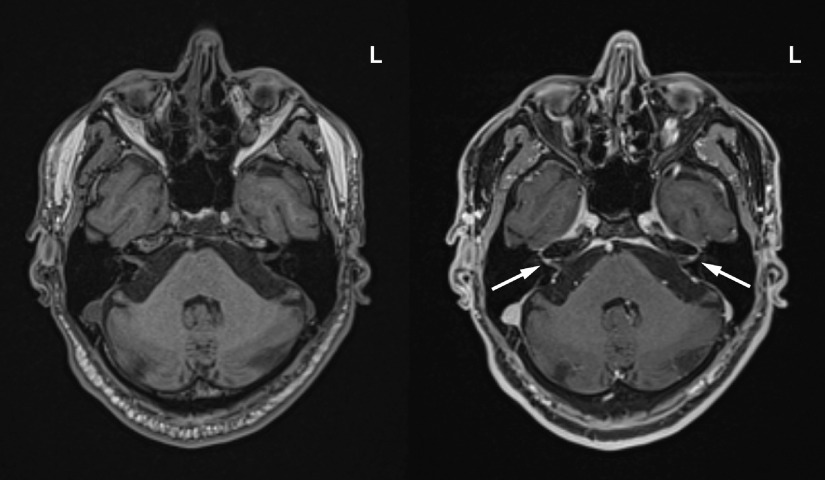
Axial T1-weighted pre-contrast (left) and T1-weighted fat-saturated post-contrast brain MRI (right) demonstrated bilateral facial nerve enhancement involving the labyrinthine segment (arrows), tympanic segment, mastoid segment, and extracranial facial nerve. L = left.
